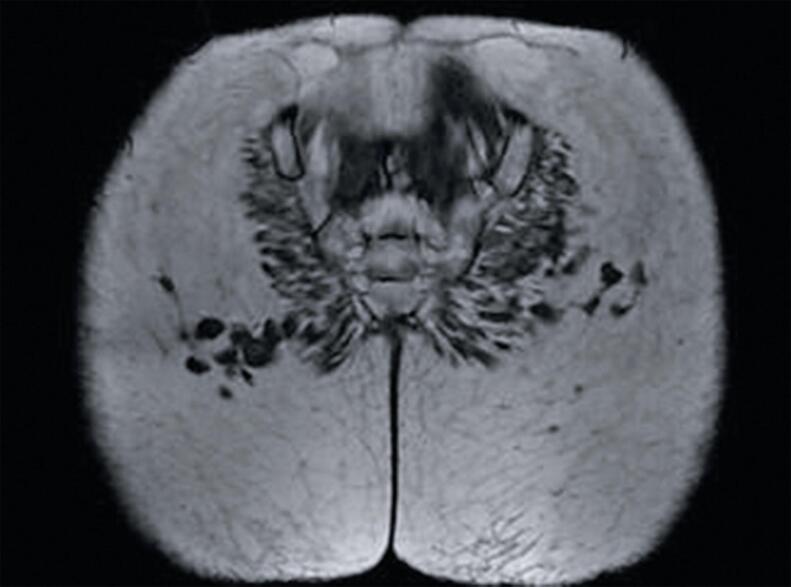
Case 2 - MRI image of gluteal granulomas
